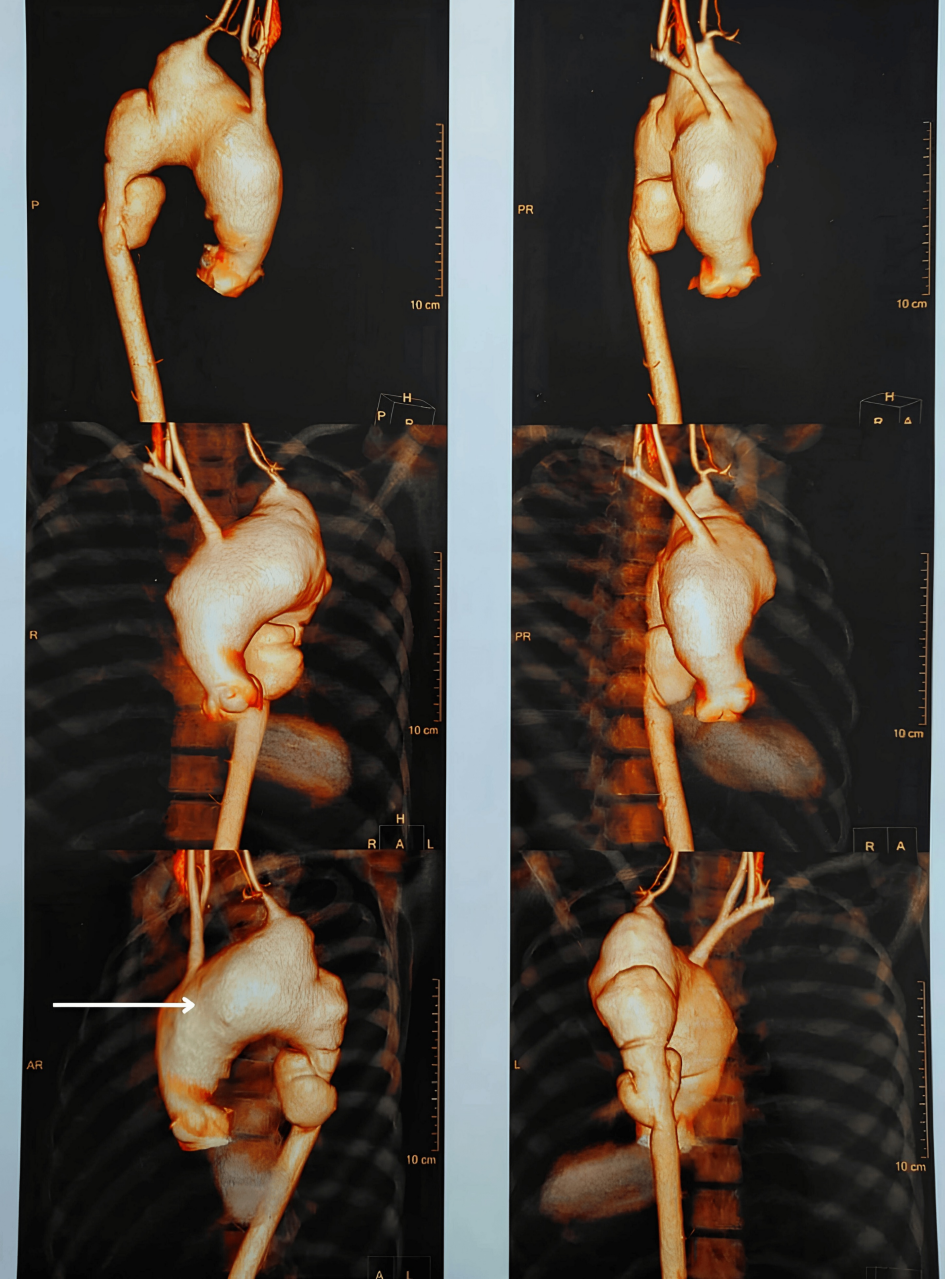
Computed tomography aortogram of the ascending aorta, arch of the aorta, and descending aorta with 3D reconstruction showing a large fusiform aortic aneurysm.
